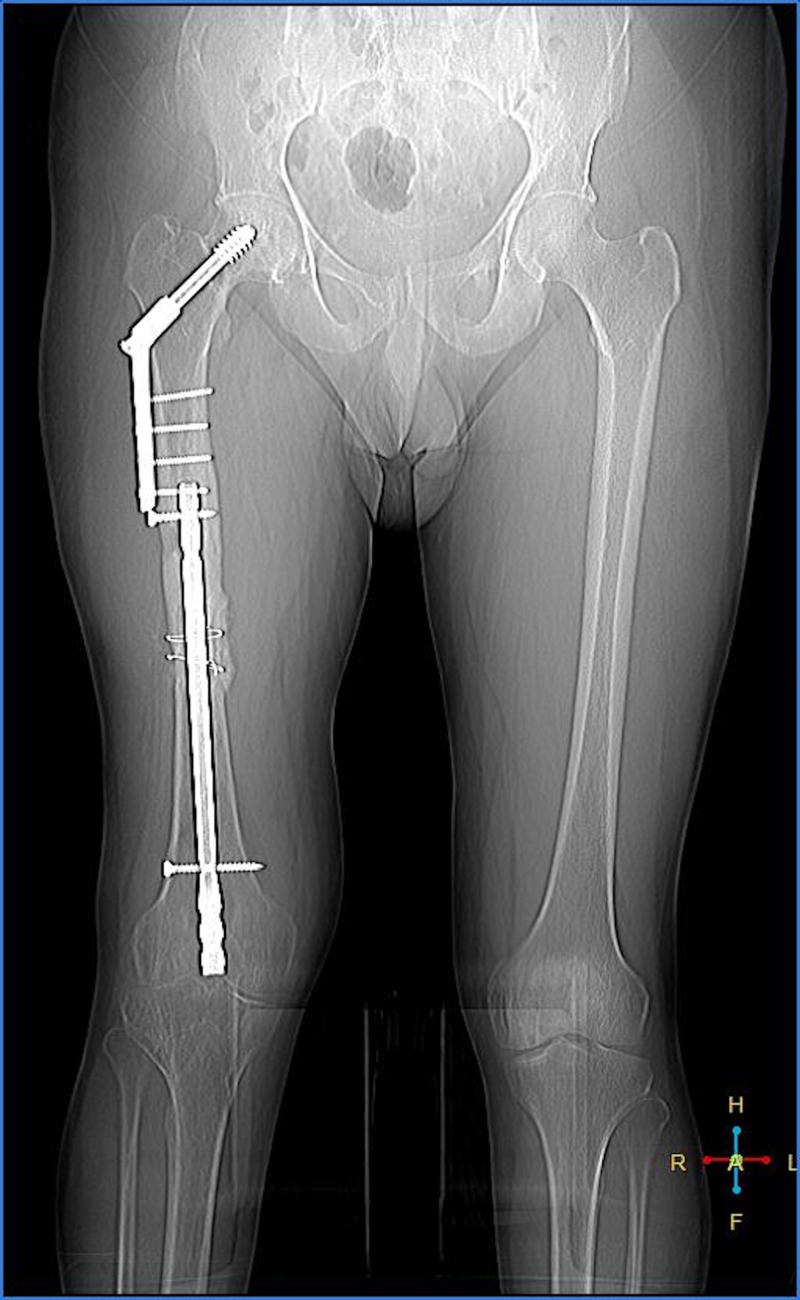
Computed tomography scanogram of the right lower limb with implants in situ
